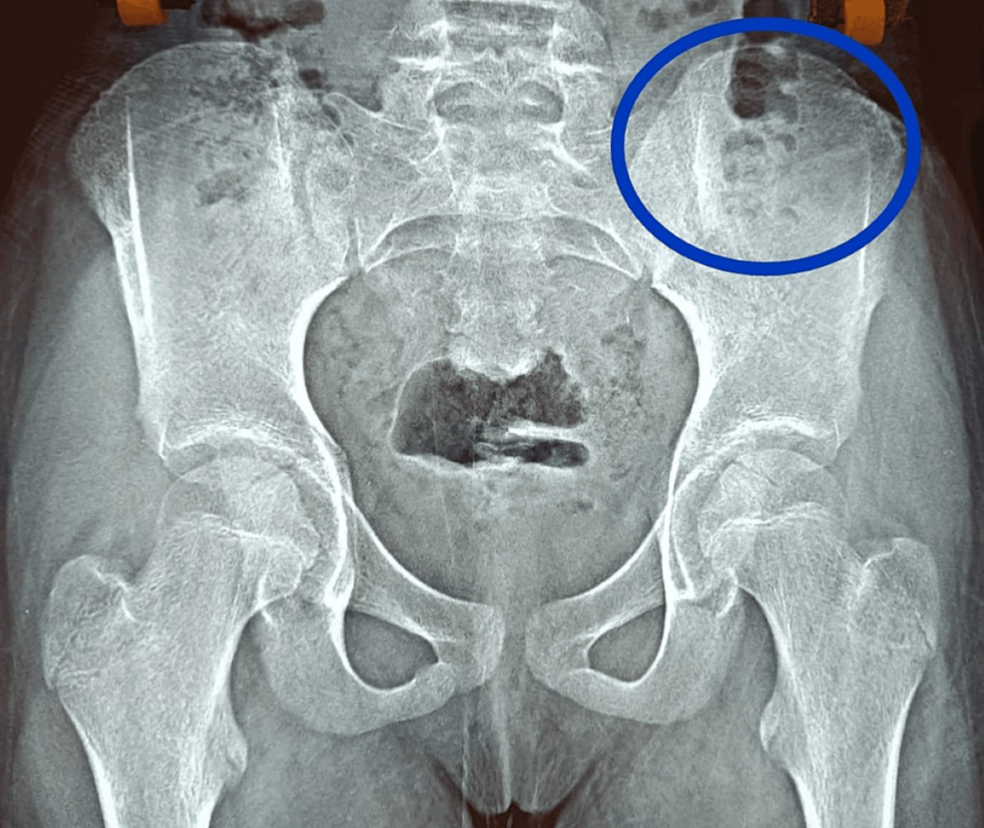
Postoperative radiograph after six months showing the integrity of iliac apophysis
